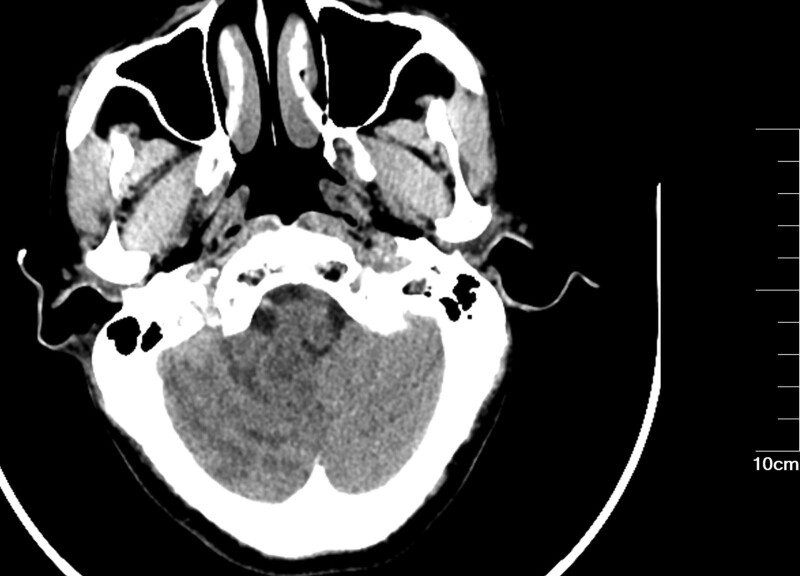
Follow-up CT 5 days later demonstrated a hypointense image of the right cerebellum (Inferior posterior cerebellar artery blood supply area). CT = computed tomography.
